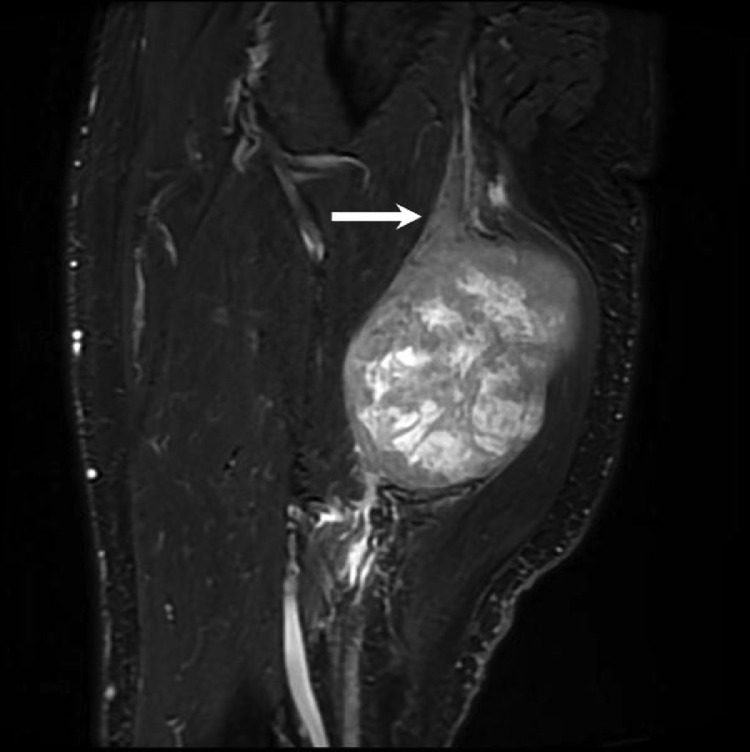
Sagittal T2 fat-saturated MRI image confirms that the mass is continuous with the sciatic nerve proximally (arrow).
